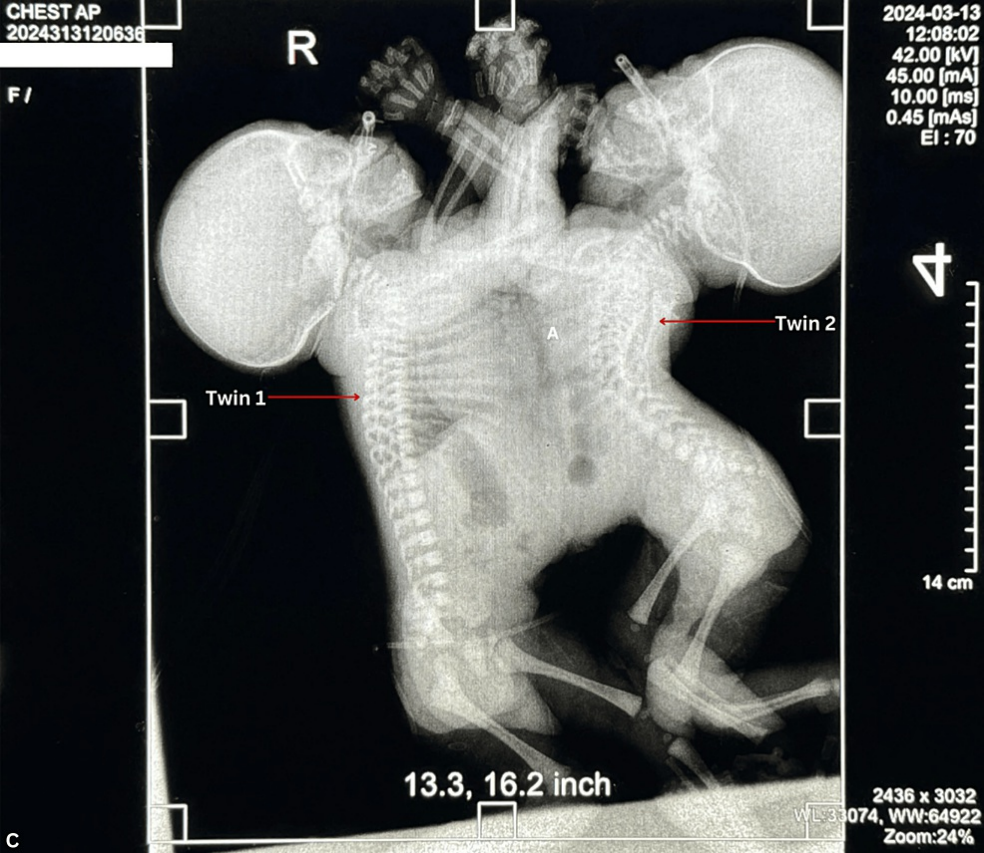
X-ray imaging of the Anteroposterior (AP) view of the thoracic cavity, demonstrating the common hepatic and cardiac structures shared by both twins, alongside their respective individual gastrointestinal tracts.
